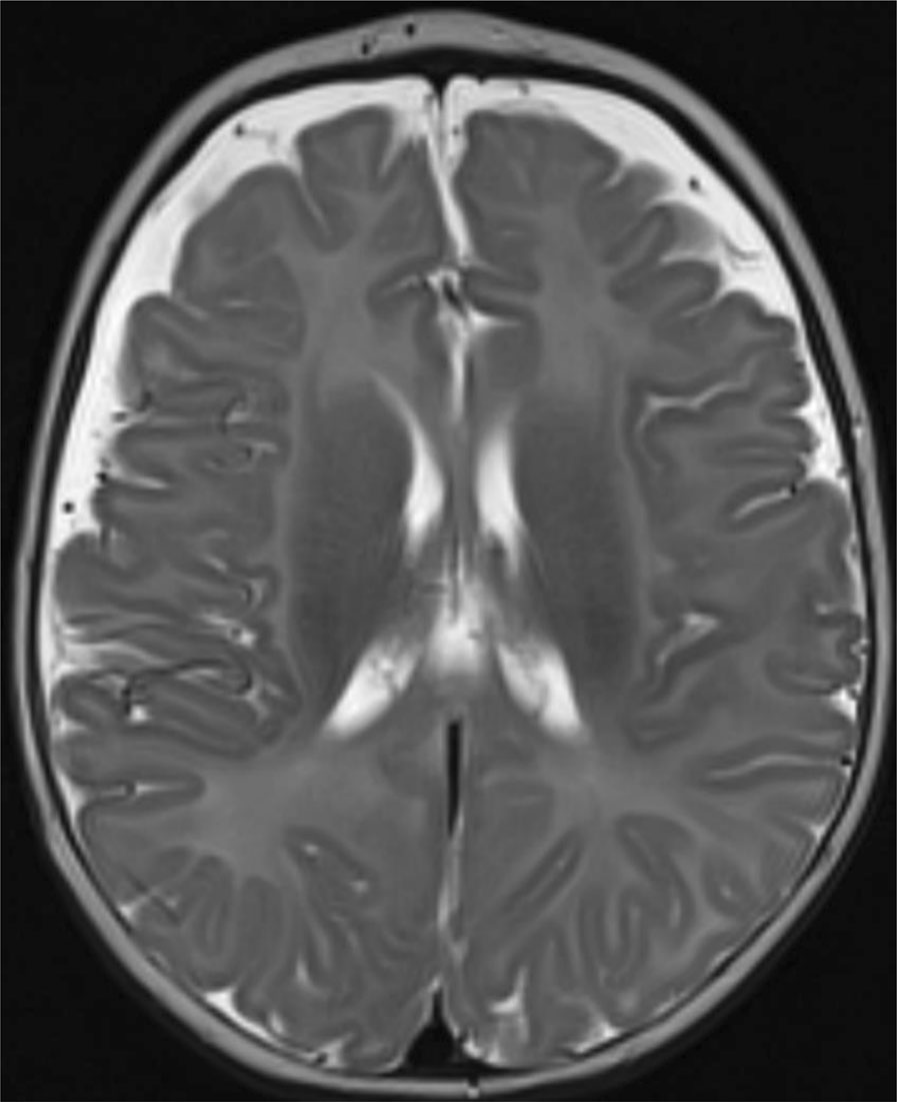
Axial FLAIR image of the brain of case 3 with CPT2D performed at four months of age, one month following her acute decompensation event. Selected image at the level of the lateral ventricles demonstrates prominence of the extra-axial CSF spaces overlying the frontal lobes. Myelin appearance is appropriate for age
